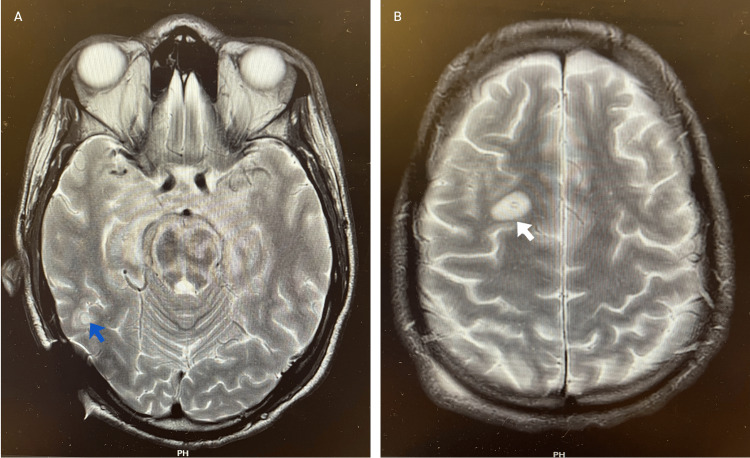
MRI head T2-weighted axial images demonstrating new lesions: (A) round hyperintense with a slight hypointense rim in the left posterior cerebrum and left-side external ventricular drain site. (B) rounded hyperintense lesion in the left frontal white matter. MRI: magnetic resonance imaging, blue arrow: lesion in the left posterior cerebrum, white arrow: lesion in the left frontal white matter
